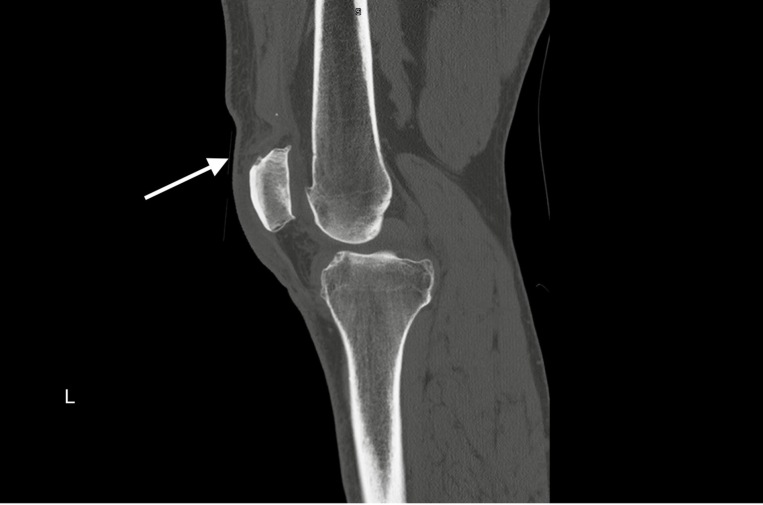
Computed tomography of left knee demonstrating near-complete tear of quadriceps tendon with retraction of the central portion of the tendon (arrow).
